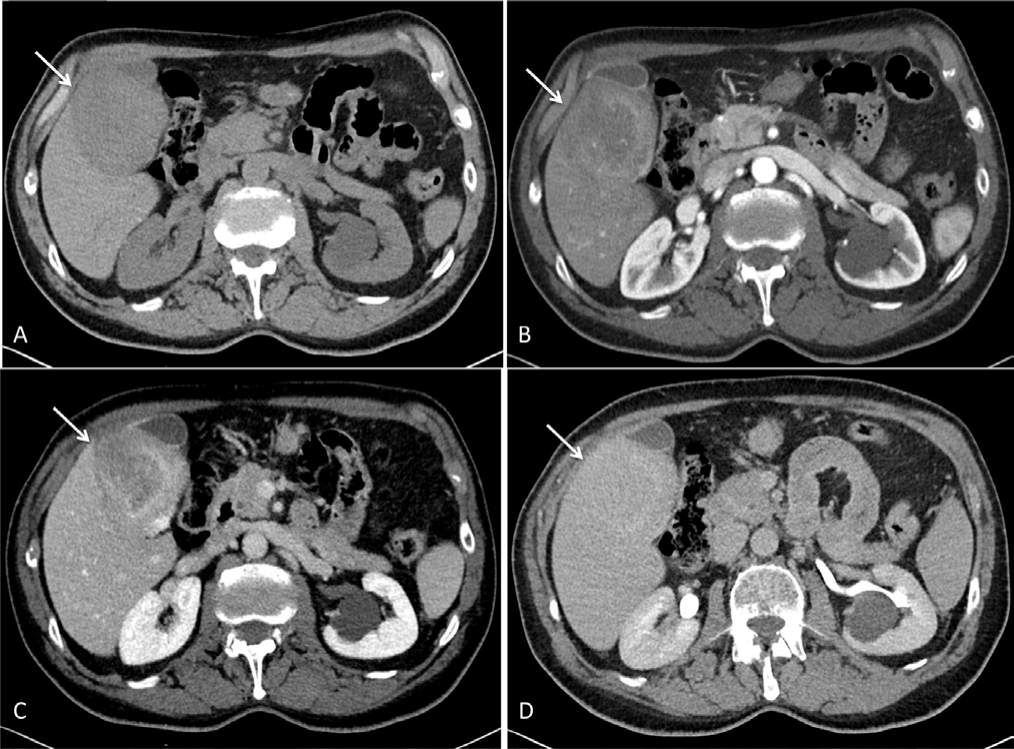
Precontrast CT image (A) shows a weak heterogeneous hypodense mass along
